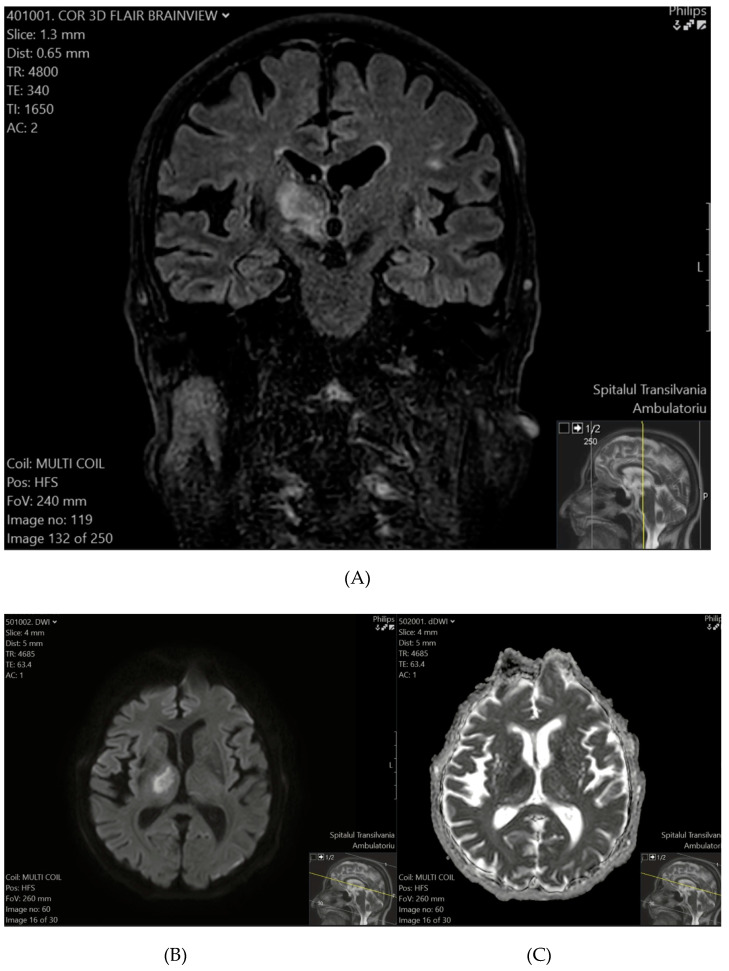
(A) (top) T2-FLAIR shows a hyperintense lesion in the right thalamus and the posterior limb of the internal capsule. (B) (bottom-left), (C) (bottom-right) DWI (B) and ADC (C) sequences confirm diffusion restriction, consistent with a recent ischemic event.
